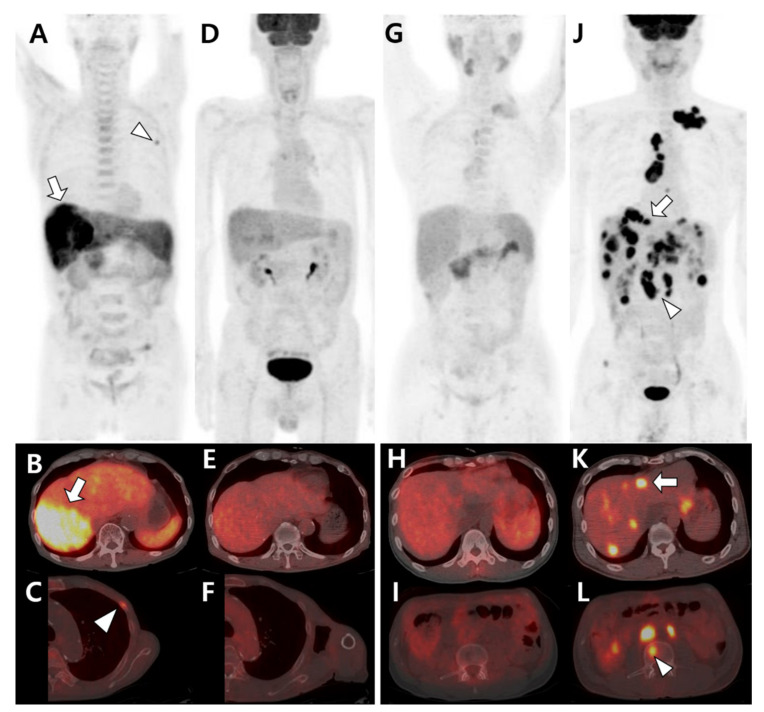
Different tracer avidities of primary and metastatic sites in patients with hepatocellular carcinoma (HCC). (A–F) A 77-year-old male patient with well-differentiated HCC. ^11^C-acetate positron emission tomography (PET)/computed tomography (CT) (A–C) shows high acetate uptake in a primary hepatic tumor (arrow in A,B) and metastatic bone lesion in the left third rib (arrowhead in A,C). However, ^18^F-fluorodeoxyglucose (^18^F-FDG) PET/CT (D–F) shows no significant uptake in the related sites. (G–L) A 43-year-old male patient with poorly differentiated HCC. ^11^C-acetate PET/CT (G–I) shows mild uptake or isometabolism in the hepatic tumor (H) and metastatic bone lesion (I). In contrast, ^18^F-FDG PET/CT (J–L) shows intense FDG uptake in hepatic tumors (arrow in J,K) and multiple metastatic lesions in the lymph nodes and bones (arrowhead in J,L).
